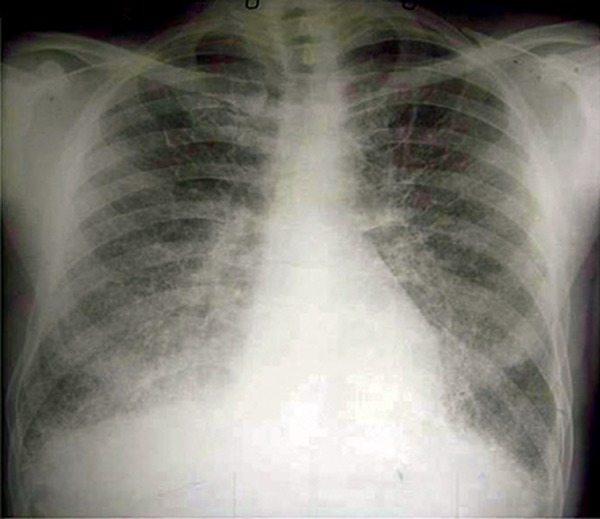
Chest X-ray: parenchyma puntiform shadows bilaterally diffuse in lungs, predominantly in the middle and lower fields.
